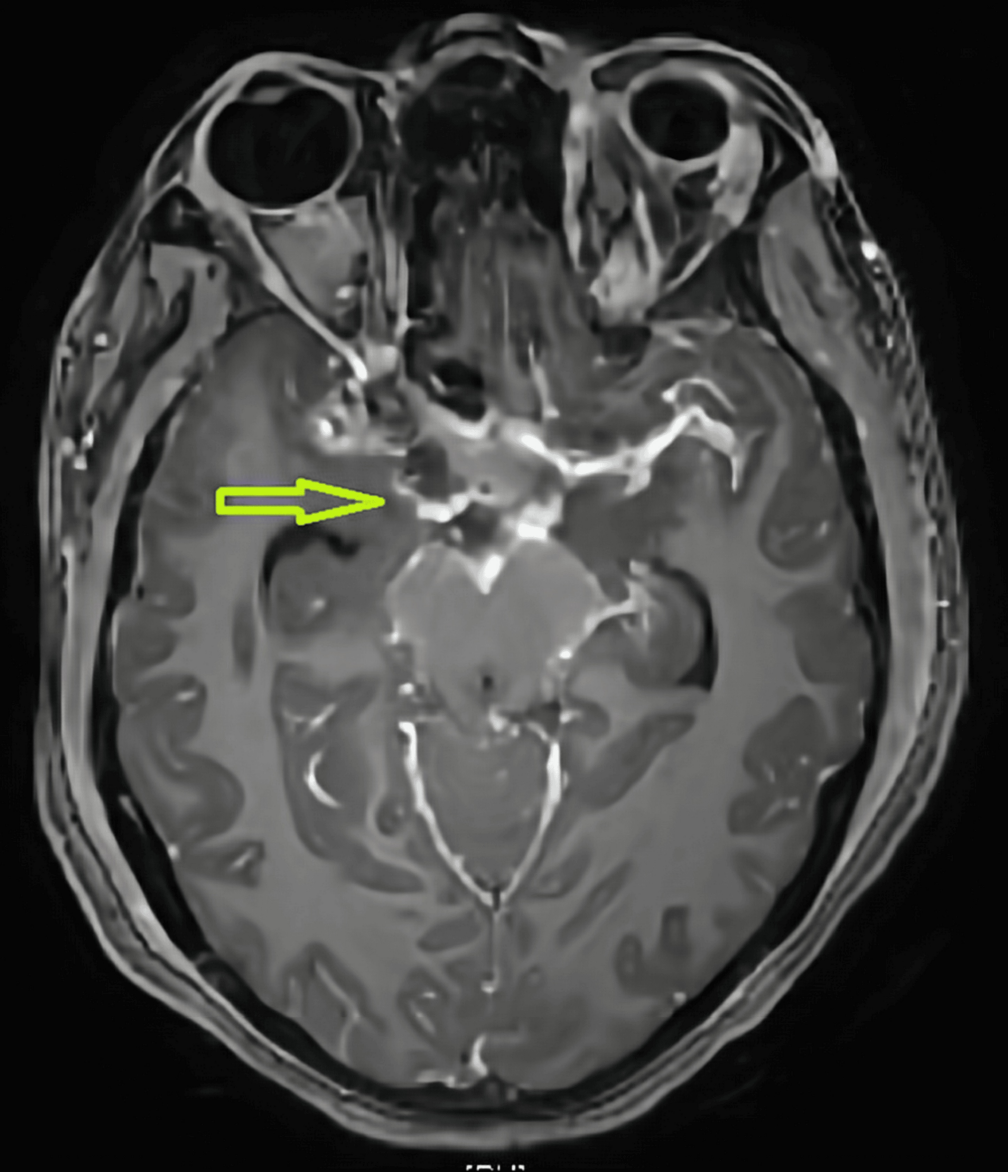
Axial T1-weighted post-contrast (gadolinium-enhanced) MRI image showing lepto-meningeal enhancemnt
